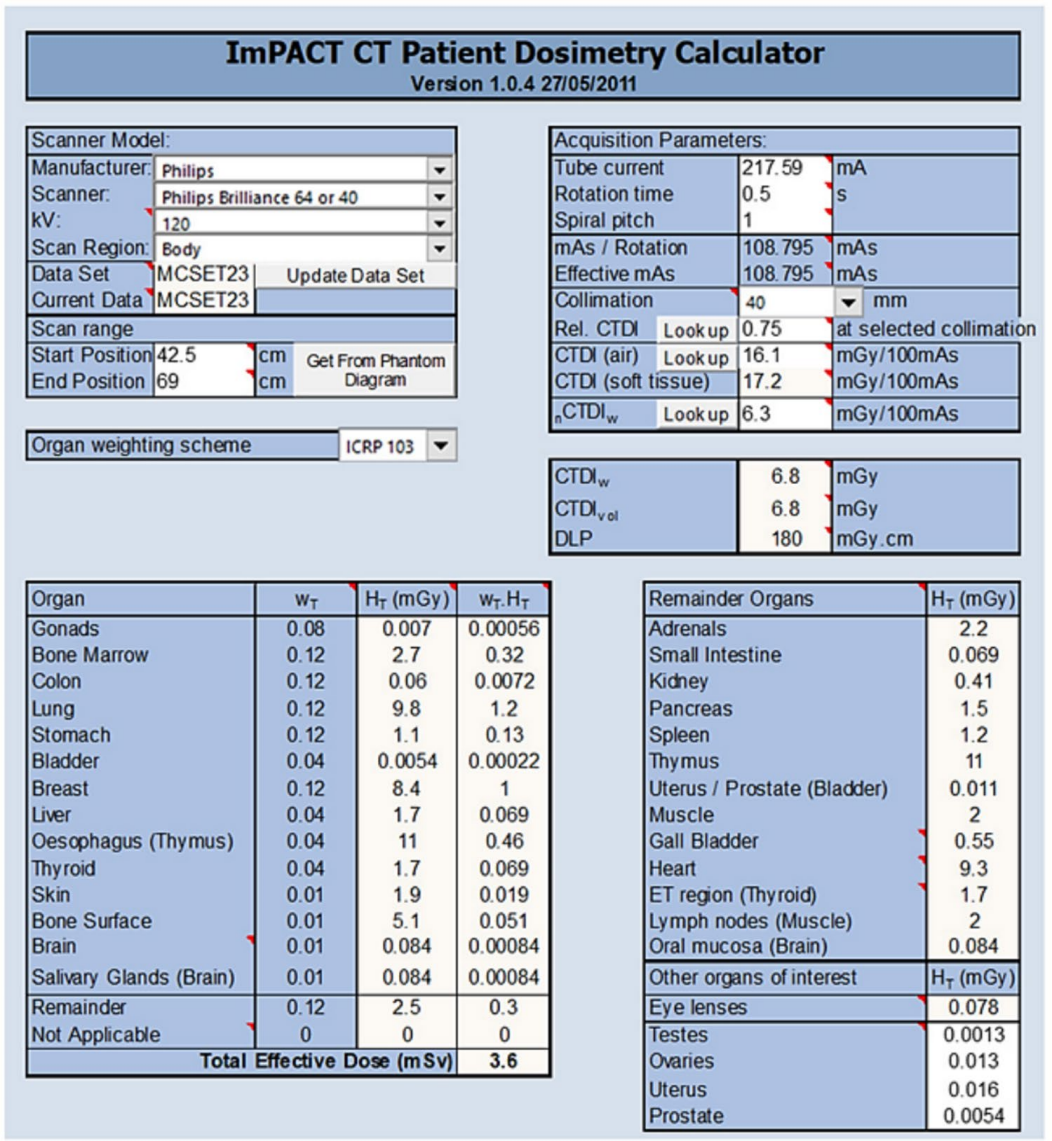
Overview of the ImPACT CT dosimetry spreadsheet used for estimating organ doses and effective radiation dose
